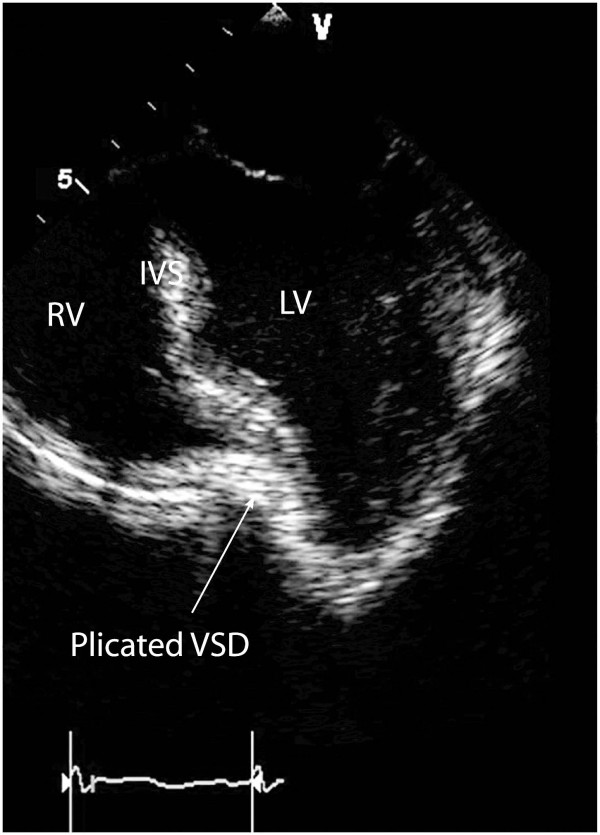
Mid-esophageal, 4 chamber, 2D Transoeophageal echocardiogram showing septal plication. IVS = interventricular septum.
